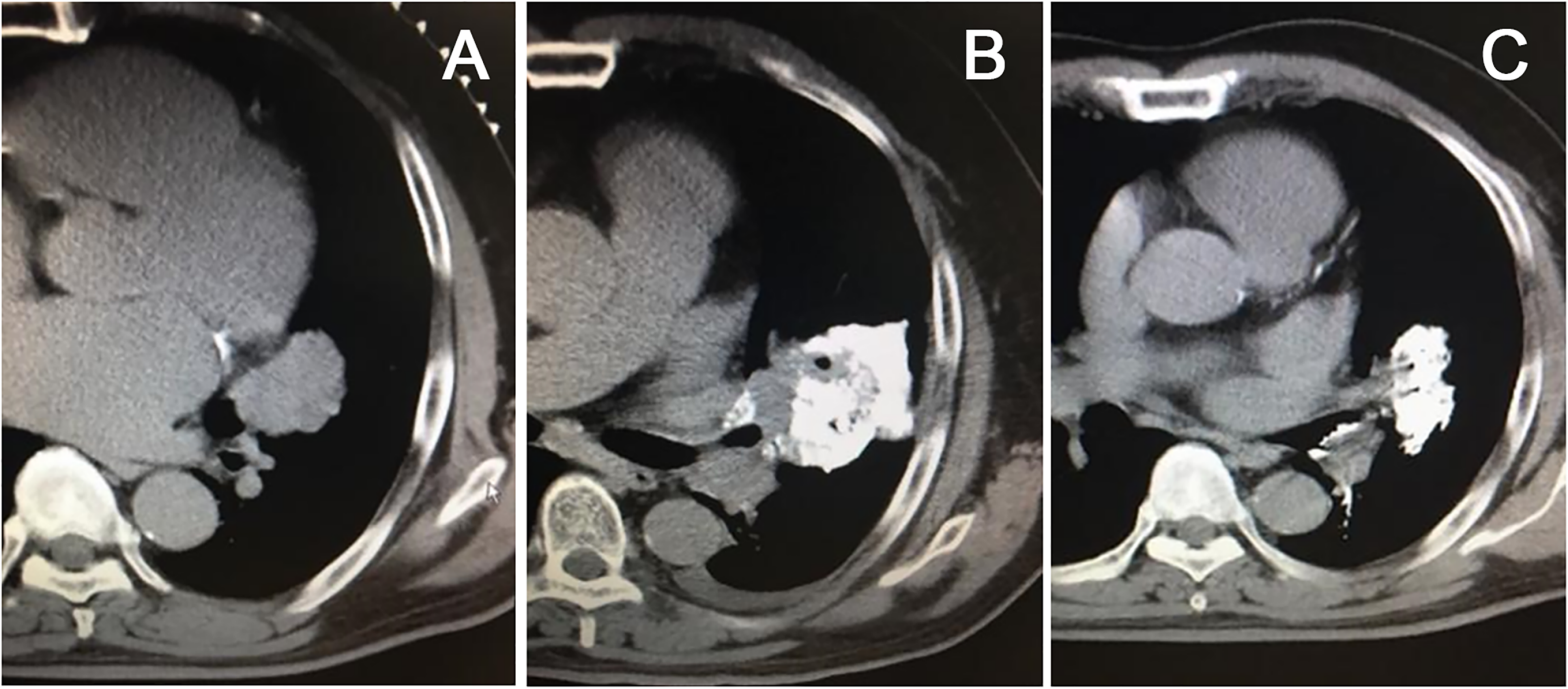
Chemical ablation of left-sided central lung cancer, CT scan showed a large lesion in the hilar region (A), chemical ablation was performed with cisplatin plus epiamphetamine, and iodinated oil was used for tracing (B). The mass shrank significantly three months after treatment (C).
